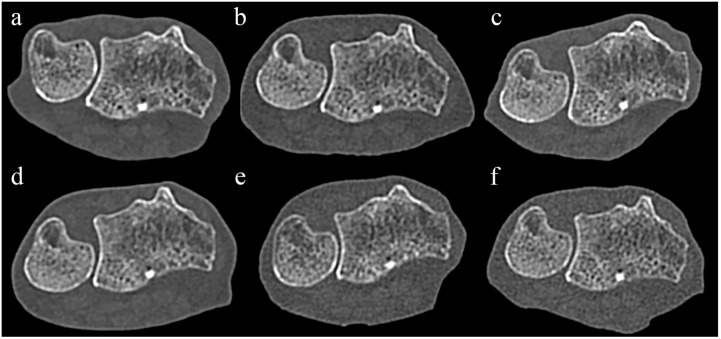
Comparison of photon-counting CT (PCCT) and energy-integrating-detector CT (EIDCT) images in the standard (STD) mode. Images were obtained using (a) Alpha, (b) Drive, (c) X.cite, (d) Force, (e) AS, and (f) Flash scanners. Qualitative scores were made using the image (b) as a reference. Although the appearance of cortical bone and trabecular bone in each image is similar, the PCCT image (a) has less noise than the EIDCT images (b–f).
